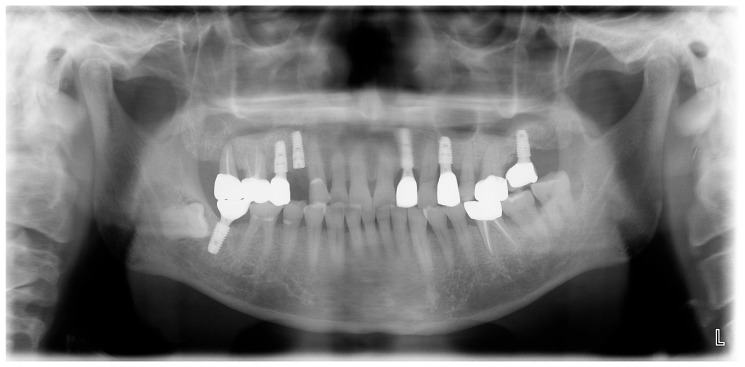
Initial panoramic dental X-ray. The implant fixture is placed in the alveolar bone of the upper right first premolar, and the external part has been removed.
